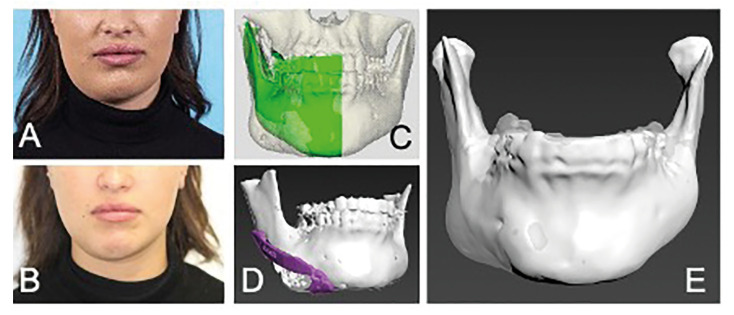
A 15-year-old patient with DSO/TP of the right mandible. (A + B) Pre- and post-operative pictures, on which a decrease in swelling of the right mandible can be observed. (C + D) 3D reconstruction of CBCT-scan on which the osseous swelling of the right mandible can be seen. In green a mirrored model of the mandible to determine the extent of asymmetry and to determine the resection osteotomy with matching patient-specific surgical guides. (E) 3D reconstruction of CBCT-scan pre- (transparent) and postoperative, showing a more symmetrical appearance d.
